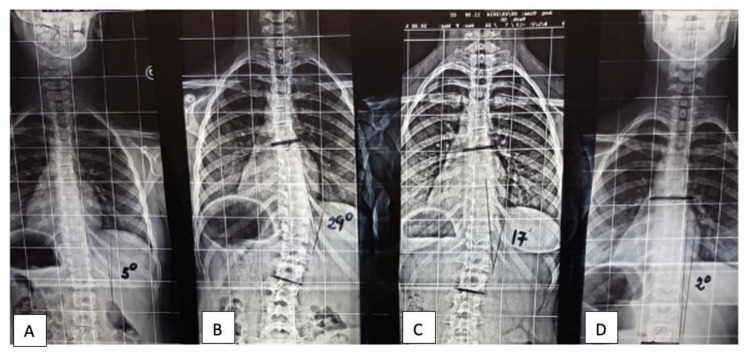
X-ray of the spine of a patient before and after rapid expansion of the palatal suture (RPE). (A) Cobb 5° Risser 0%. Without appliance. (B) Cobb 29° Risser 20%. 6 months after RPE device application. (C) Cobb 17° Risser 40%. 6 months after the removal of RPE device. (D) Cobb 2° Risser 60%. 12 months after the removal of RPE device.
